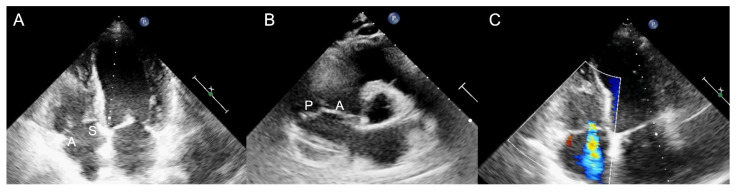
Transthoracic echocardiogram in two dimensions. (A) Apical four-chamber view: the septal leaflet (S) is seen adjacent to the septum and the anterior leaflet (A) adjacent to the right ventricular free wall; (B) Parasternal short-axis view: the anterior leaflet (A) is visualized next to the aorta and the posterior leaflet (P) on the opposite site, adjacent to the right ventricular free wall; (C) Apical four-chamber view with color-Doppler documenting tricuspid regurgitation (white arrow).
